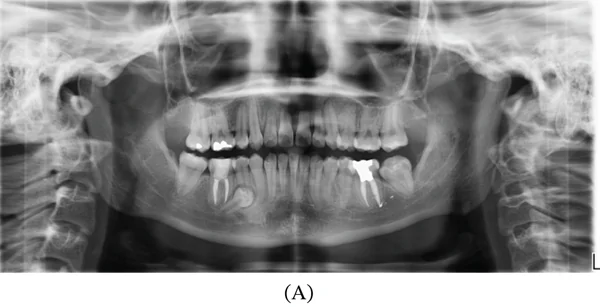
(A) Case #2 posttreatment panoramic radiograph.
